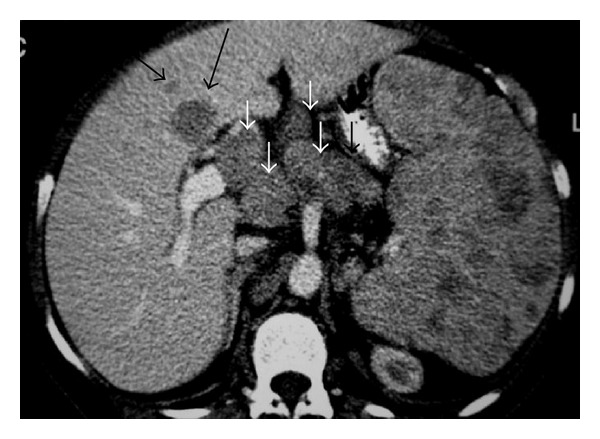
NHL. Axial contrast-enhanced CT image shows multiple low-attenuation splenic nodules. Note multiple enlarged LN (small arrows) and hepatic lesions (long arrows).
